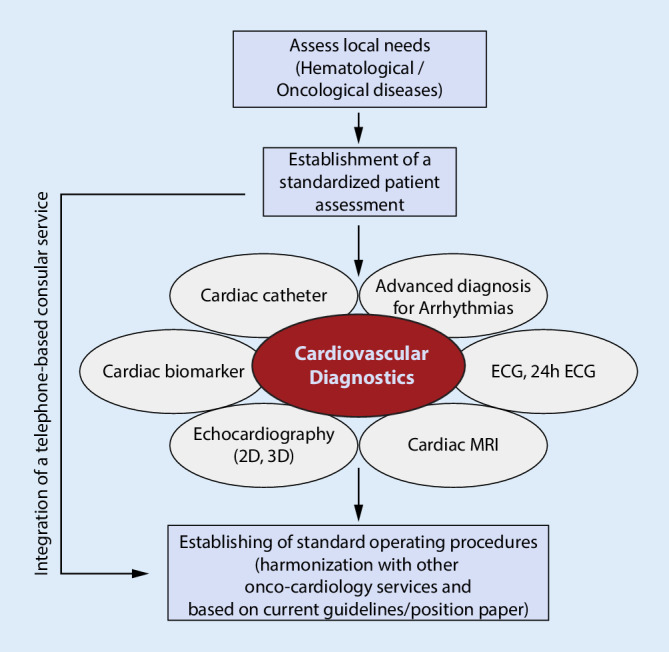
Adaptation to local needs and integration of diverse cardiac diagnostic needs are necessary. For early stratification of patients, an additional telephone-based consultation service might be helpful for larger clinical centers. *MRI* magnetic resonance imaging
